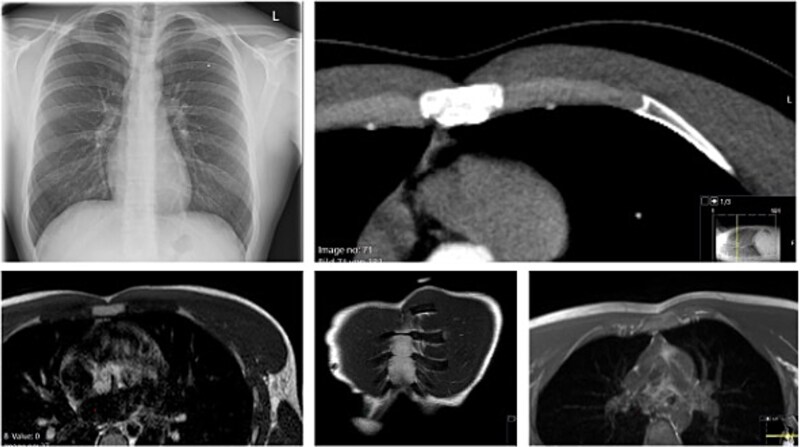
Preoperative imaging with X-ray (left, top) not showing any pathological finding. CT scan reveals a parasternal non displaced rupture of the cartilage. The MRI (bottom three images) proof the rupture of the cartilage and the swelling of the periosteum.
